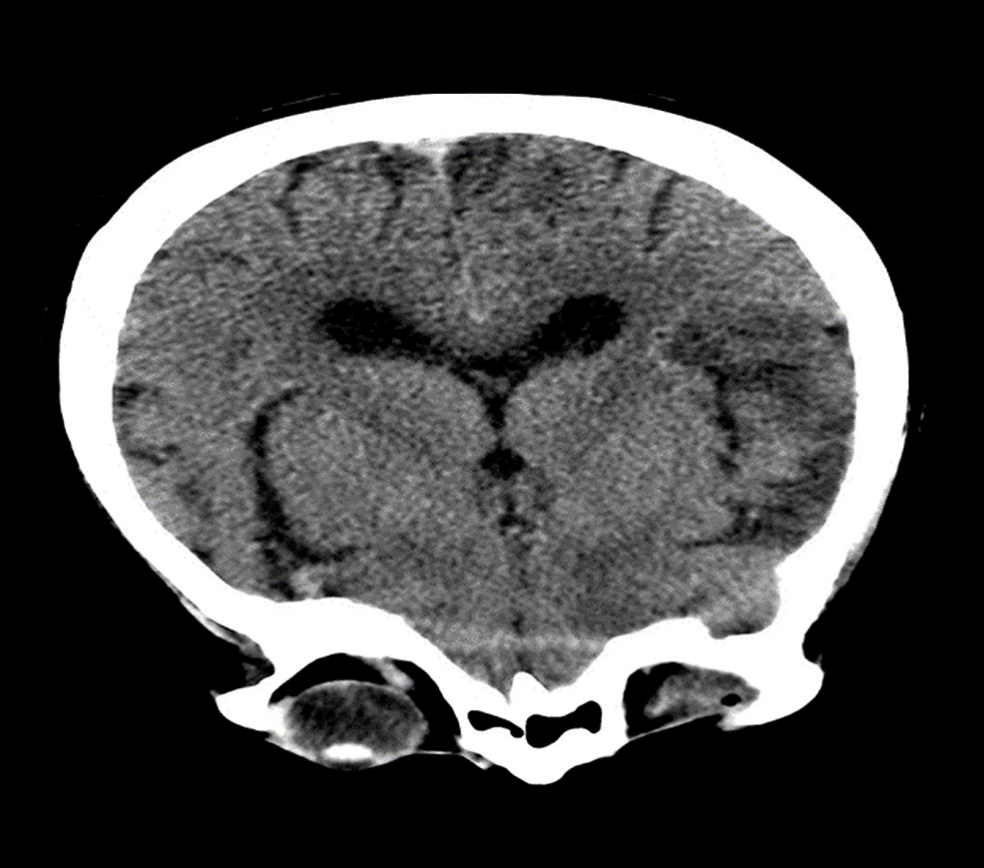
CT brain on the first day of admission Brainstem and cerebellum without evidence of focal lesions. Ventricles are normal size. Midline is straight.
